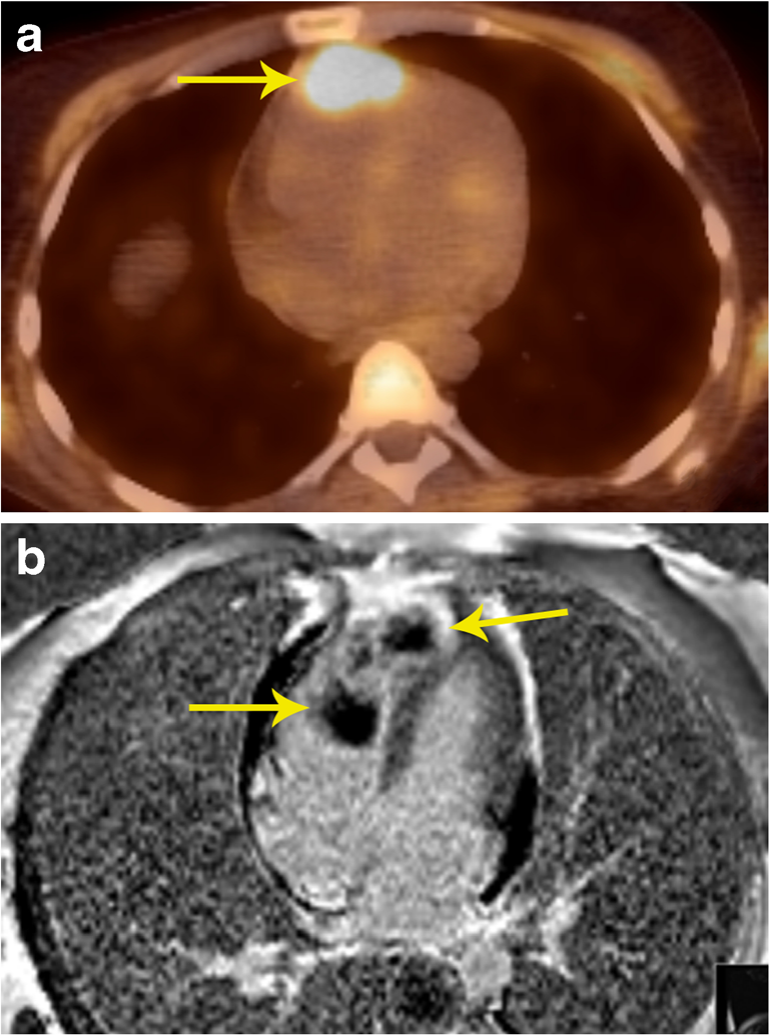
Localization of abnormal activity. a FDG-PET/CT image in a patient with breast cancer shows intense uptake in the anterior mediastinum abutting the heart. The exact location and extent of this lesion is not evident. b Axial delayed enhancement image shows that there is a heterogeneously enhancing mass in the right ventricle (arrow) that is extending to the right ventricular apex and also invading the right ventricular free wall
